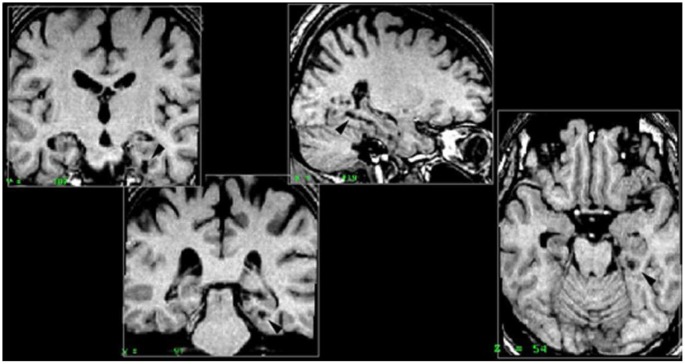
Right parahippocampal lesion. MRI section in a coronal plane, zooming-in on the lesion to the right parahippocampal cortex (RPH) of patient PP (indicated with the arrow). This section was taken 30 mm posterior to the anterior commissure (*Y* = −30) in Talairach standard stereotaxic space (Talairach and Tournoux, 1988).
